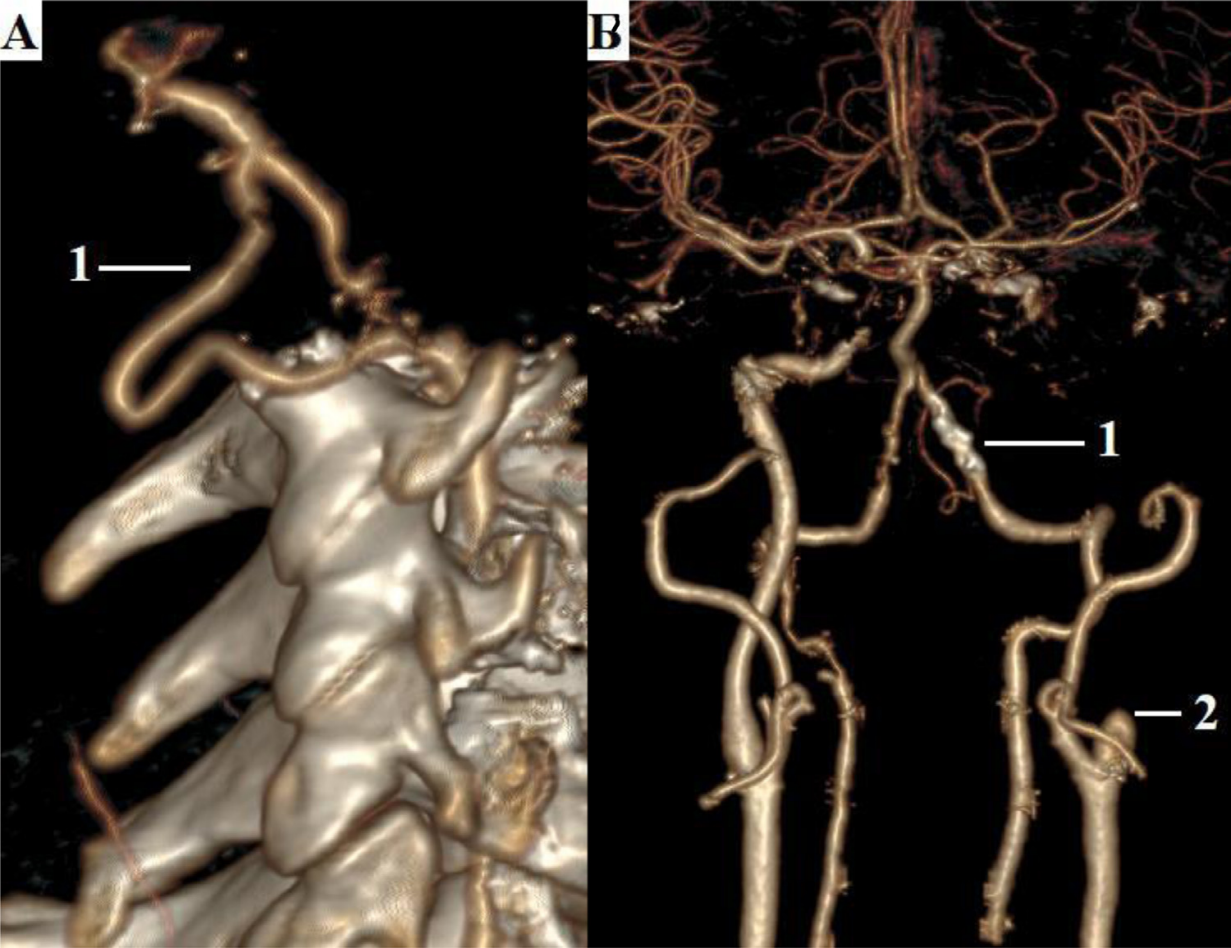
Computed tomography and angiography of extra- and intracranial arteries. (A) Lateral projection: 1 - vertebral triangle of Dr A.N. Kazantsev. (B) Direct projection: 1 - zone of thrombosis of the left vertebral artery; 2 - occlusion of the left internal carotid artery.
